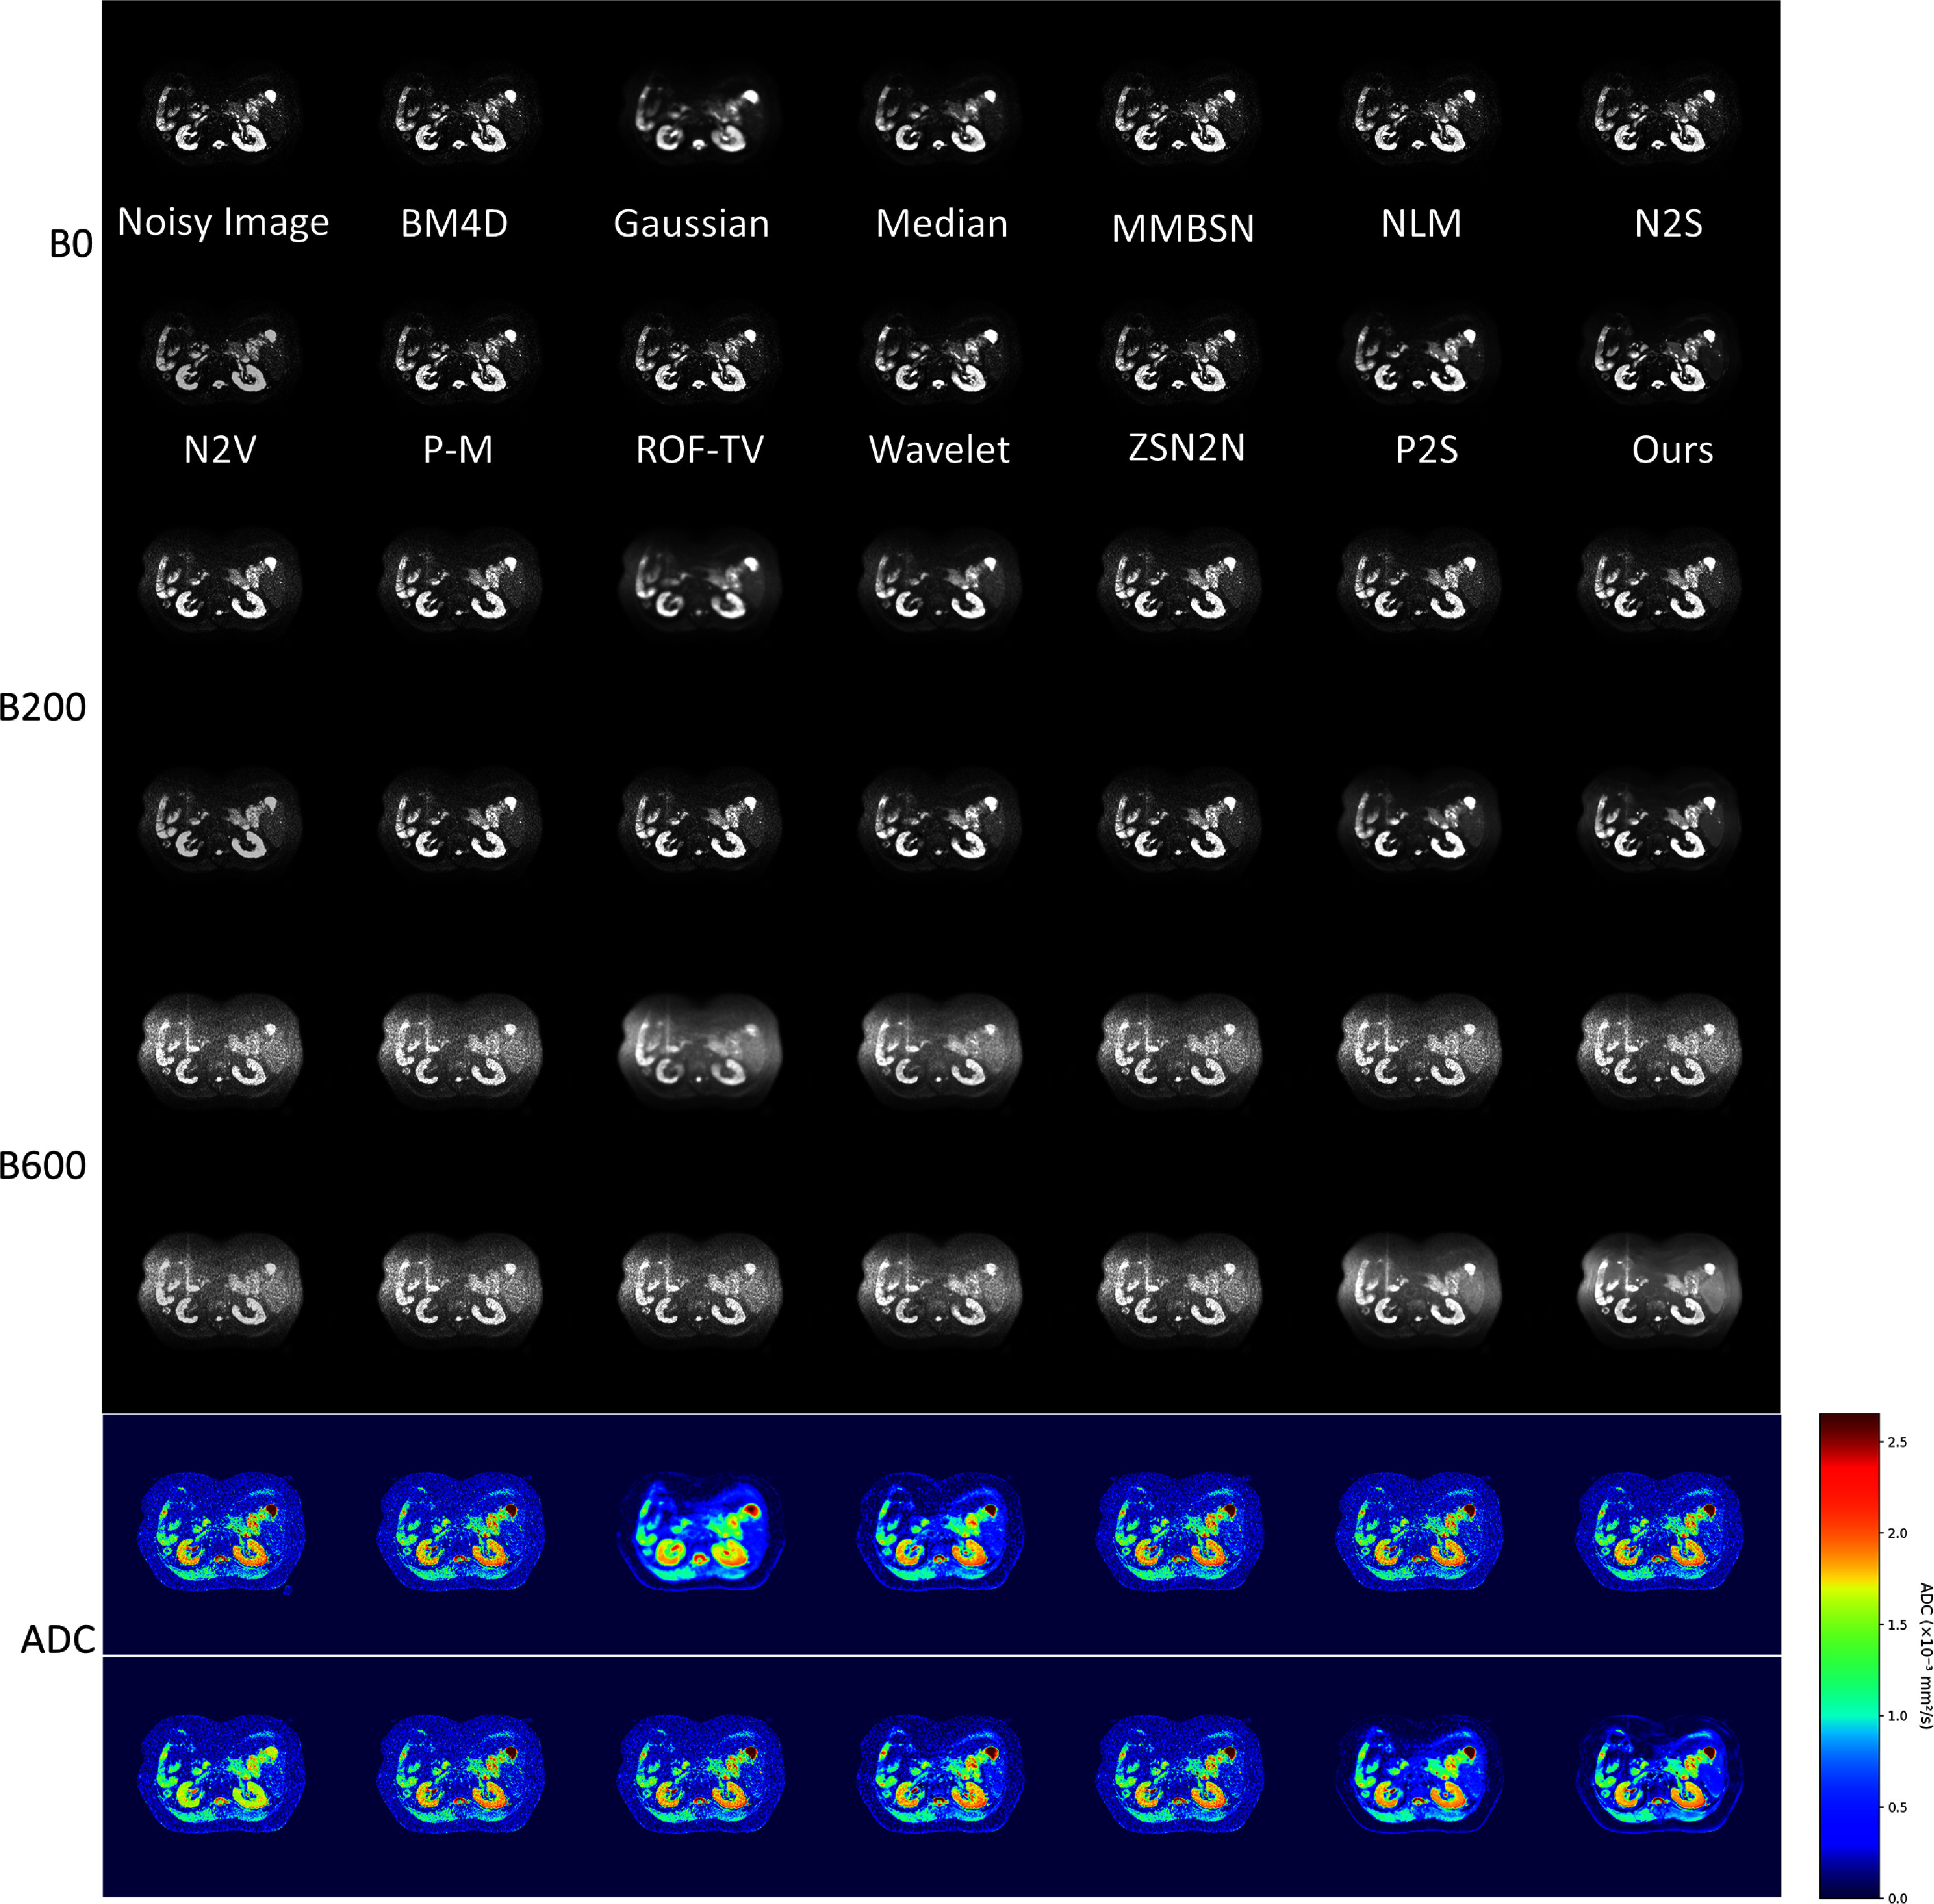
Comparison of abdominal DWI denoising results. Abdominal imaging faces challenges from respiratory motion and complex organ distribution, and BL-INR demonstrates robust denoising performance.
